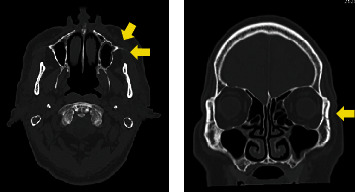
Computed tomographic images at the initial visit of a 70-year-old man with traumatic optic neuropathy (TON). Axial and coronal computed tomographic images show a fracture in the lateral wall of the left orbit. The anterior and posterior walls of the left maxillary sinus are fractured and slightly depressed. No obvious optic canal fracture can be seen.
